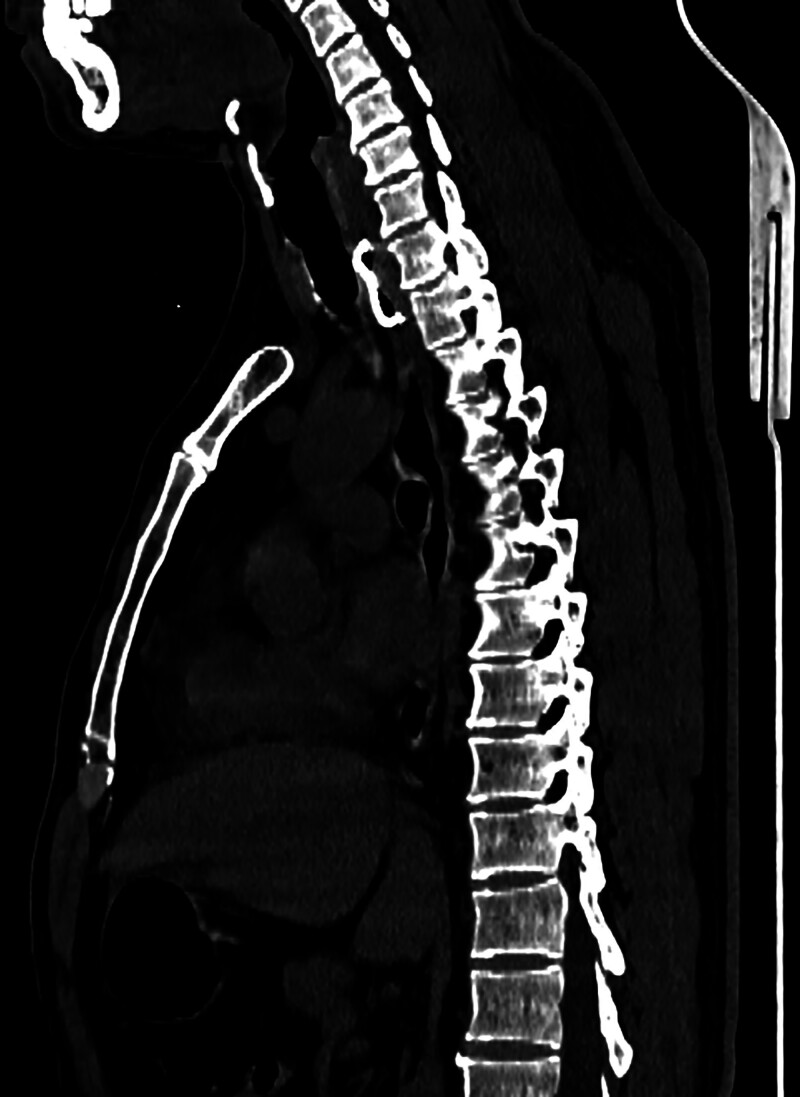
Axial, sagittal, and coronal computed tomography images revealed the foreign body to be round and flat, located slightly to the left of the anterior margin of the T1–T2 vertebral (arrow).
